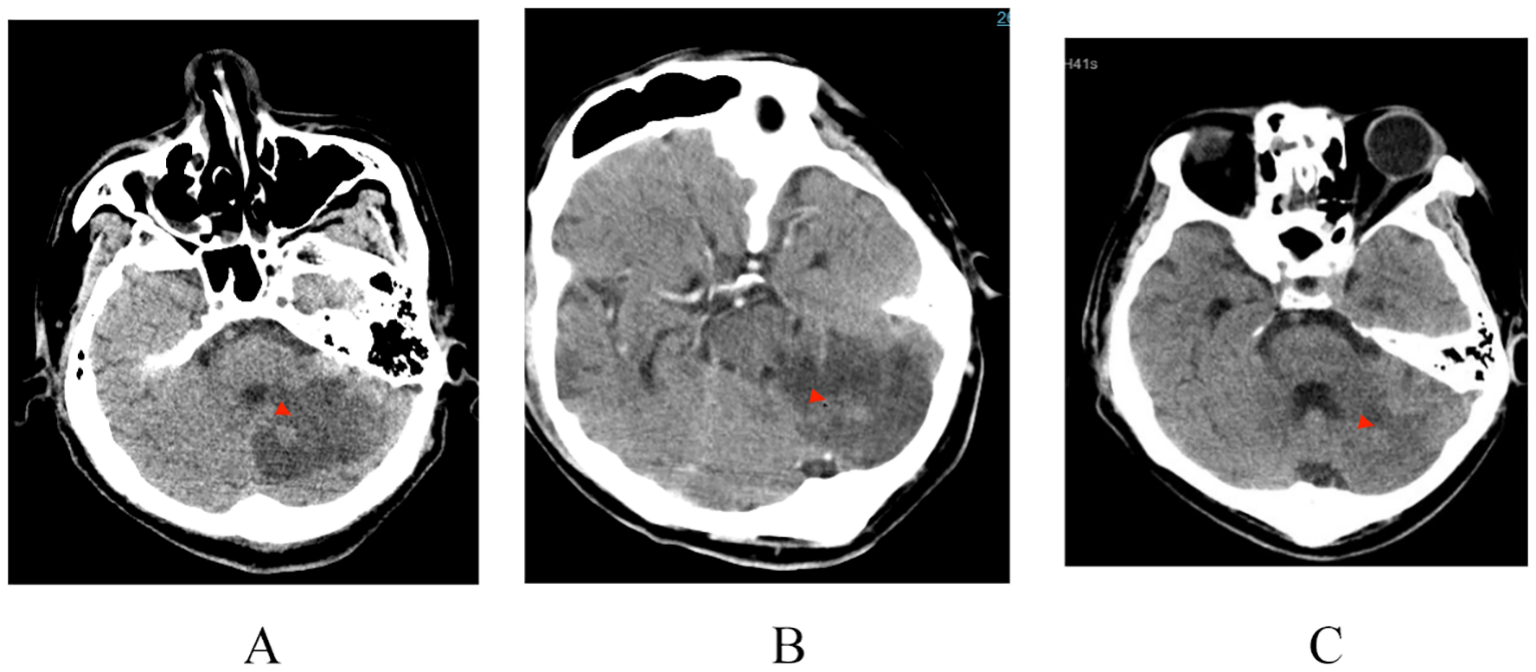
Bacteria migrate to intracranial abscess formation, gradually absorbed and improved after anti infection treatment. Cranial CT scans dated on (A) April 20 (B) April 26 (C) May 6.
